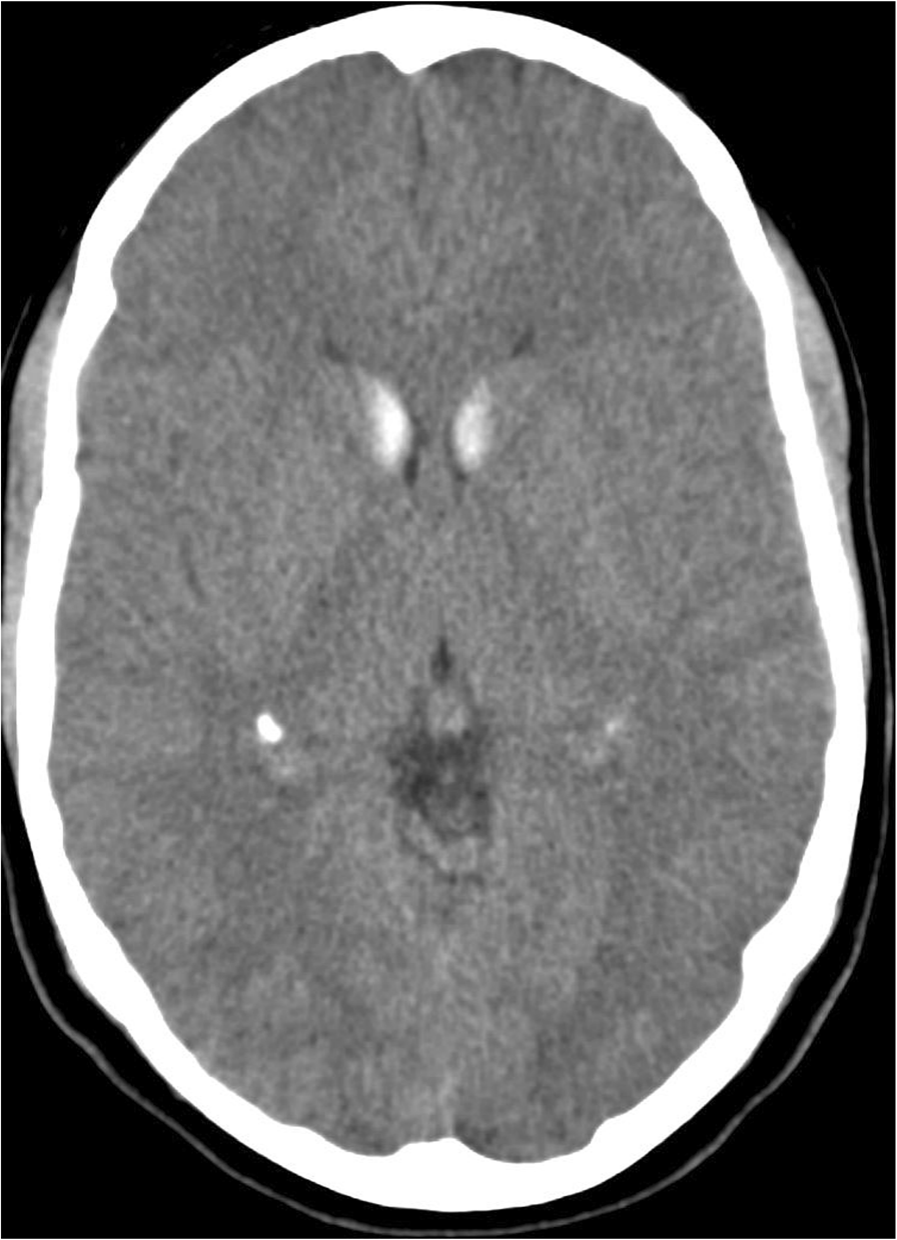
Computed tomography section of the patient showing bilateral symmetrical conflated calcifications involving the head of the caudate nucleus and thalamus
